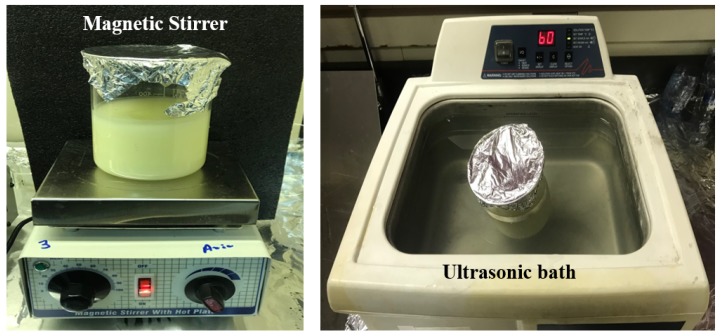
Magnetic stirrer and ultrasonic bath used to disperse Al_2_O_3_ nano-additives in the vegetable oil.
